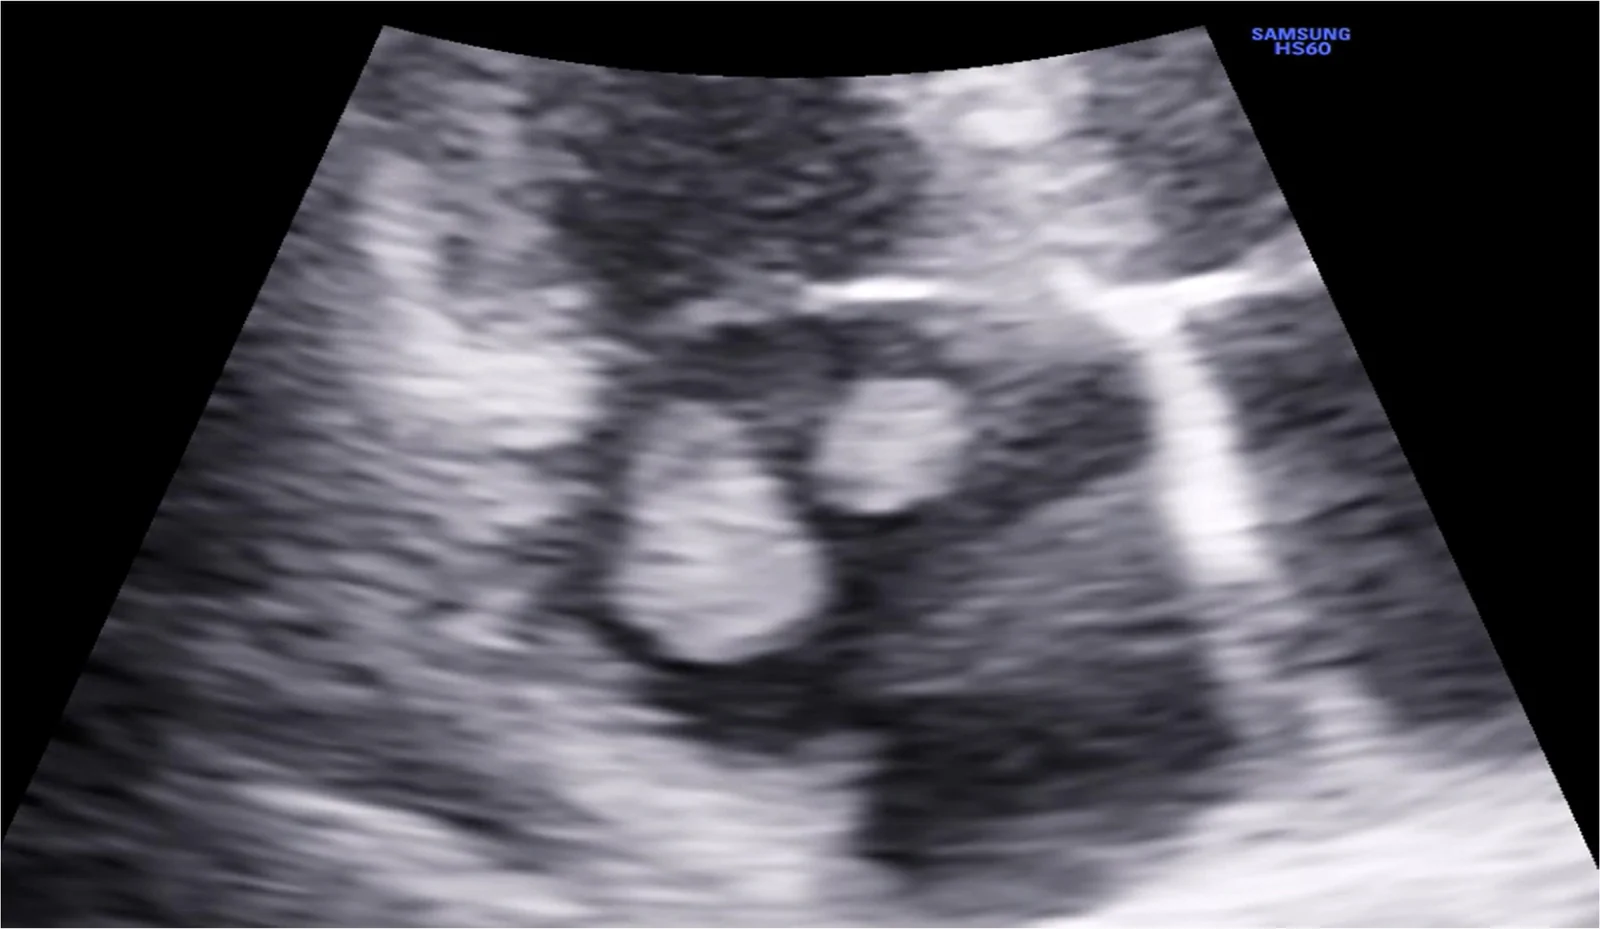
Focused transthoracic echocardiography (apical 4-chamber view) showing a heterogeneous, mobile echogenic mass within the right atrium (RA), protruding toward the tricuspid valve (TV), consistent with Behçet-related intracardiac thrombosis.
